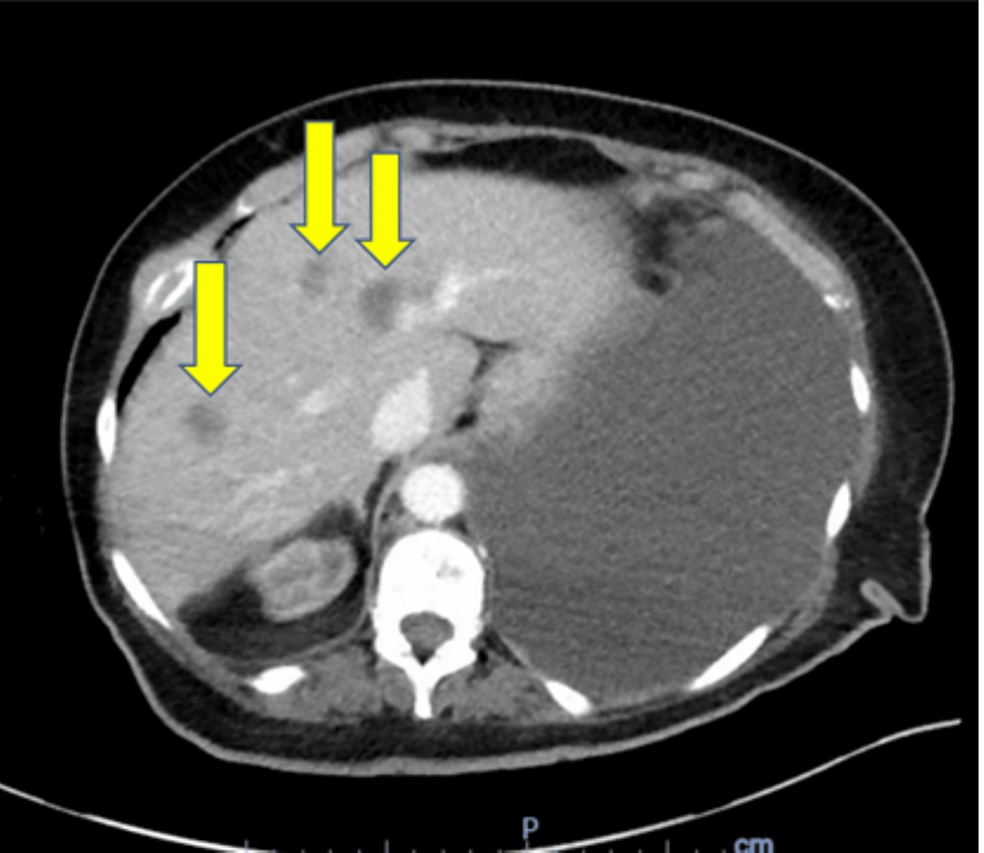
Abdominal CT showing multiple metastatic lesions (arrows)
